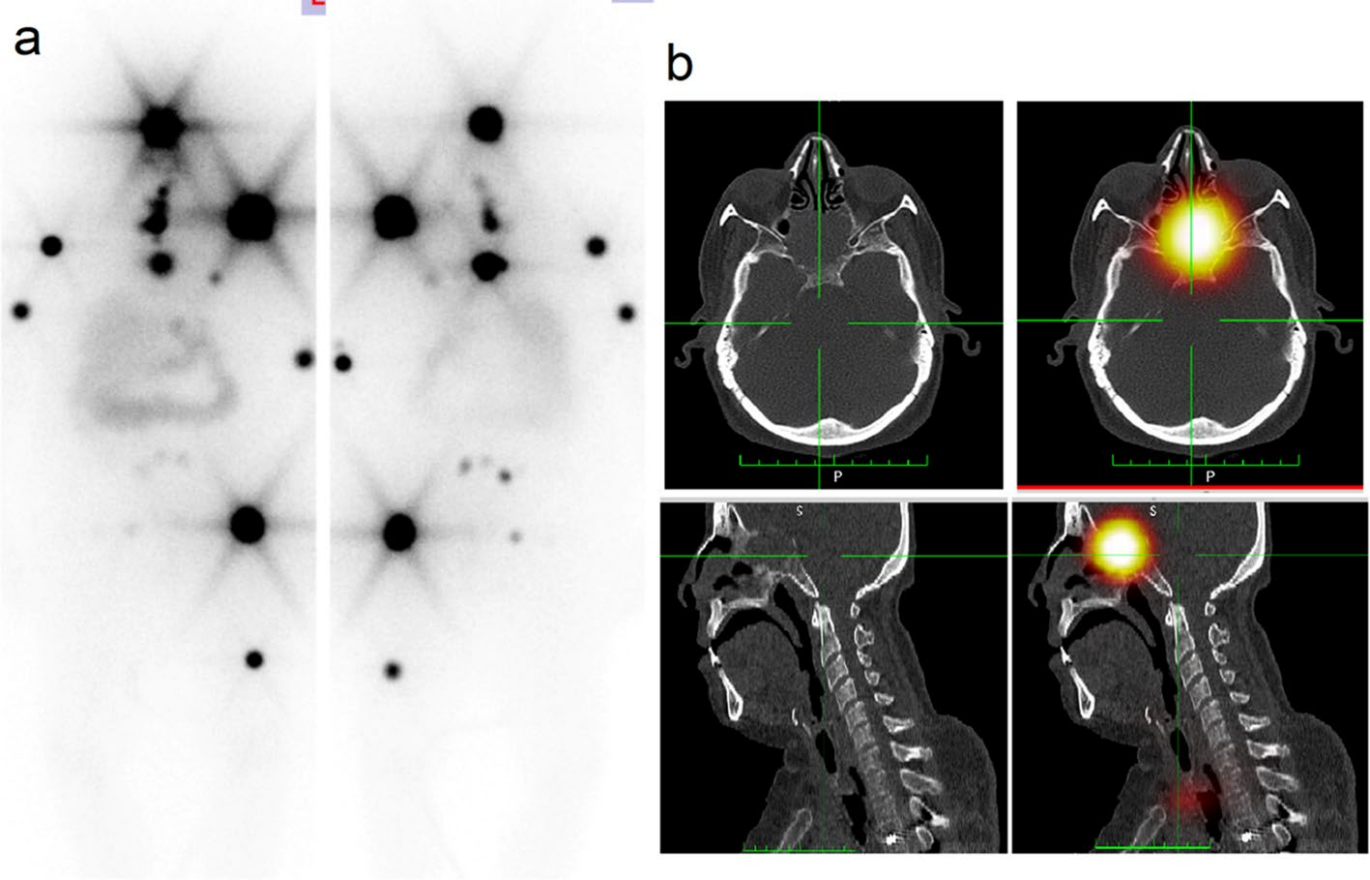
a WBI scan showed residual functioning thyroid tissue with multiple iodine avid skeletal metastases and b SPECT/CT images showed iodine avid destructive lytic metastatic lesions at sella turcica, 5th and 6th dorsal vertebrae and left humeral head, right humeral shaft and proximal end and mid shaft of left femur
